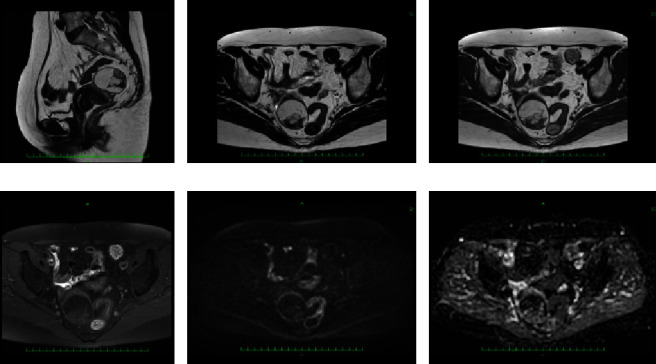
MRI at 58 years old: (a) T2-weighted image of the sagittal plane; (b) T2-weighted image of the transverse plane; (c) T1-weighted image of the transverse plane; (d) fat-suppressed T1-weighted image of the transverse plane; (e) diffusion-weighted image of the transverse plane; (f) apparent diffusion coefficient image of the transverse plane. The mass of the anterior uterine wall that was highly intense on T1- and T2-weighted images was considered a fatty component because the signal was suppressed on fat-suppressed imaging. Diffusion-weighted imaging revealed no obvious abnormal findings, suggesting that the cell density of the mass was low and was unlikely to be a malignant finding.
